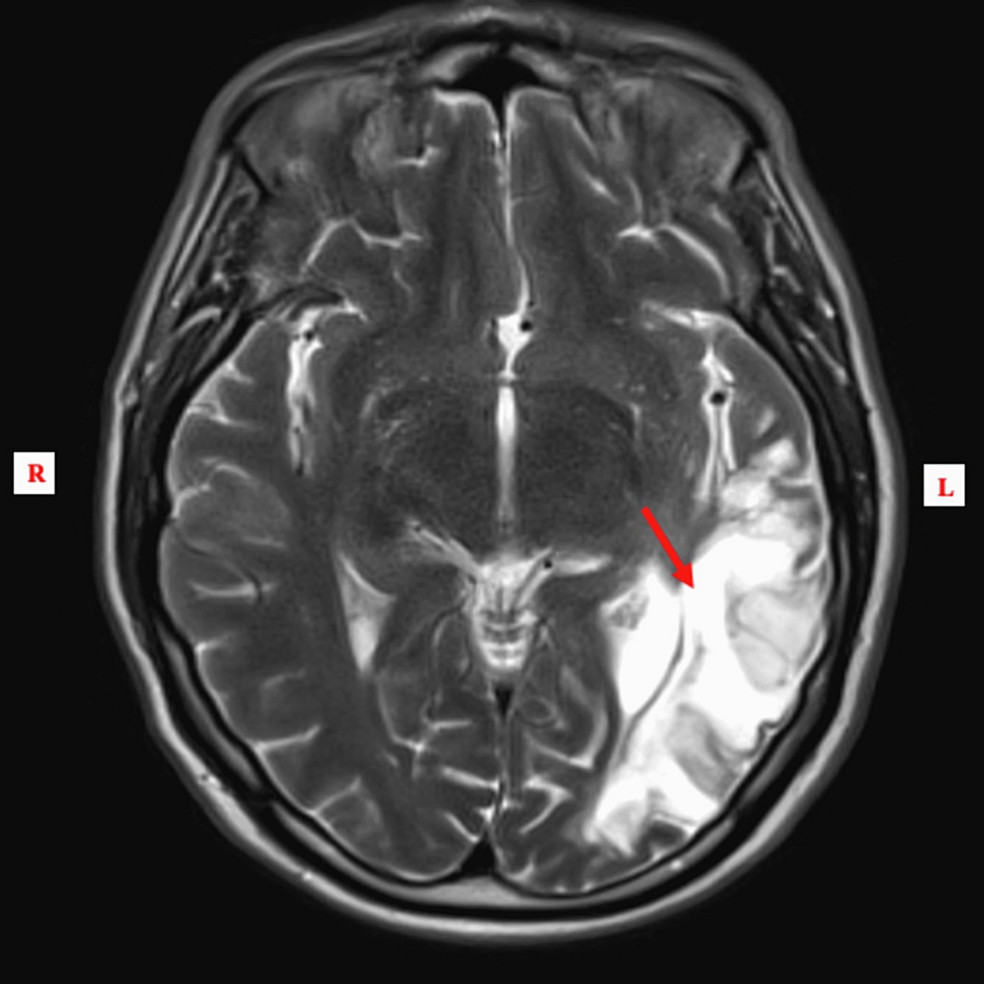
MRI with contrast (axial view) notable for a large area of encephalomalacia in the left temporoparietooccipital region
